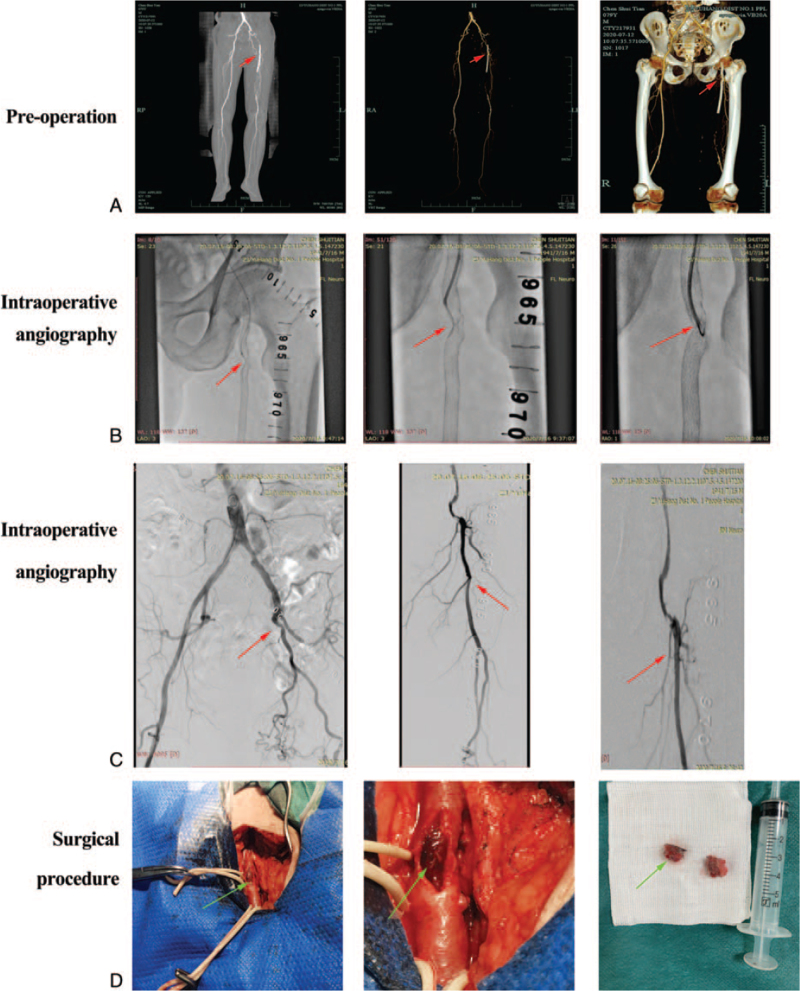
(A) Preoperative contrast-enhanced CT scan image and 3D reconstruction of the femoral artery of the lower extremity. (B) In-stent restenosis visible from intraoperative angiography. (C) Intraoperative angiography of the left side of the external iliac artery, femoral artery and femoral artery occlusion (red arrow). (D) The stenosis and rupture of the femoral artery stent may be seen once the femoral artery has been exposed.
